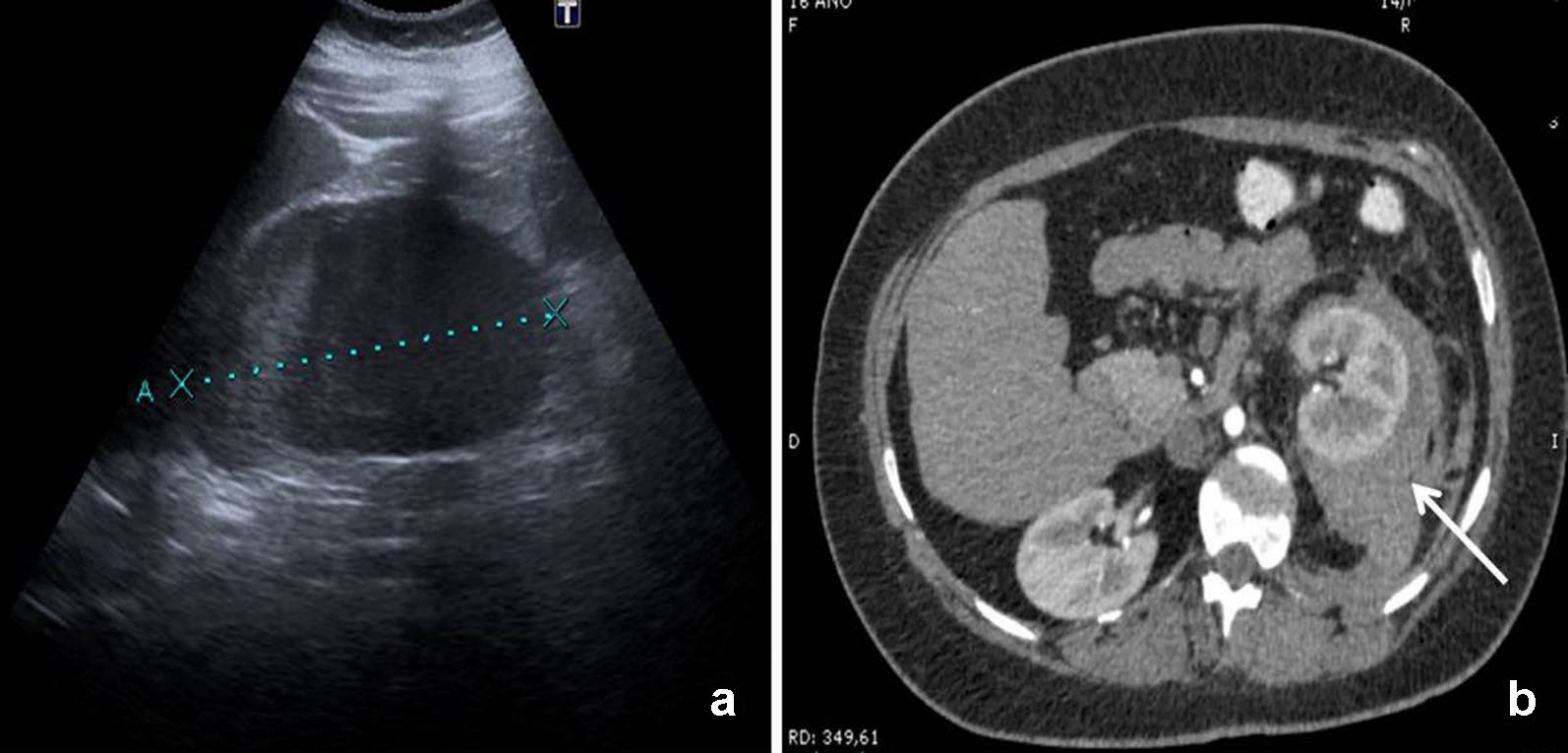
a r-EFAST: left perirenal hematoma of 1.5 cm (callipers). b CT Scan with intravenous contrast: peri- and pararenal hematoma (*arrow*) displacing anteriorly the left kidney
